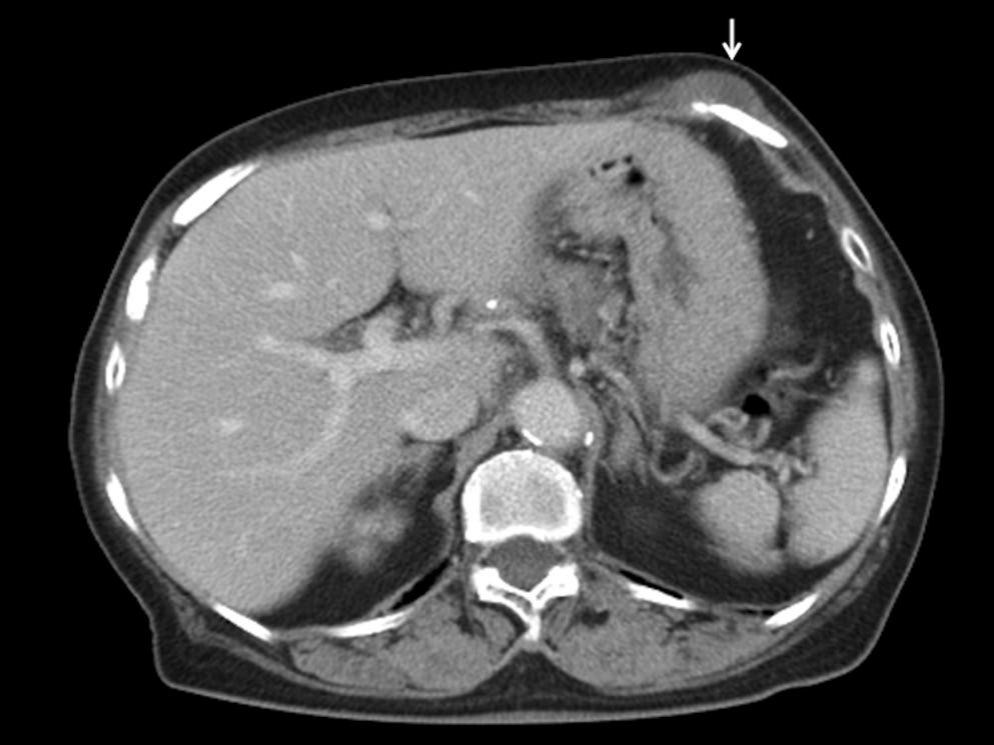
Abdominal contrast‐enhanced CT. White arrow showed pericostal mass with central hypoattenuation
